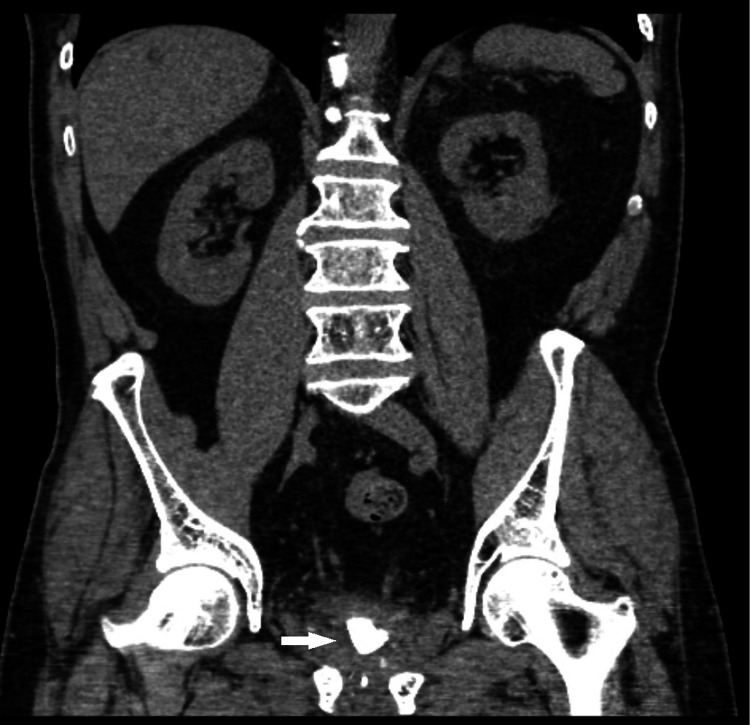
CT Abdomen coronal view depicting large bladder stone at level of bladder neck
